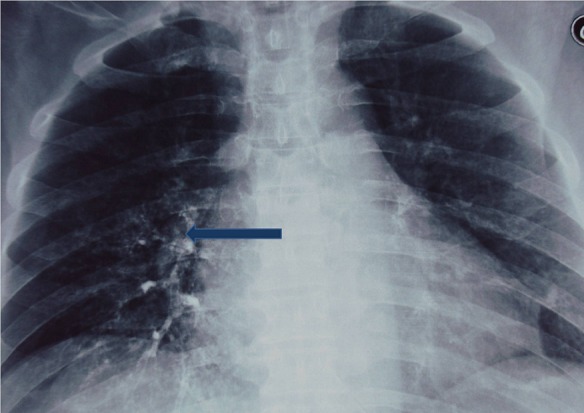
Chest X-ray showing a bronchial passage of the contrast agent
